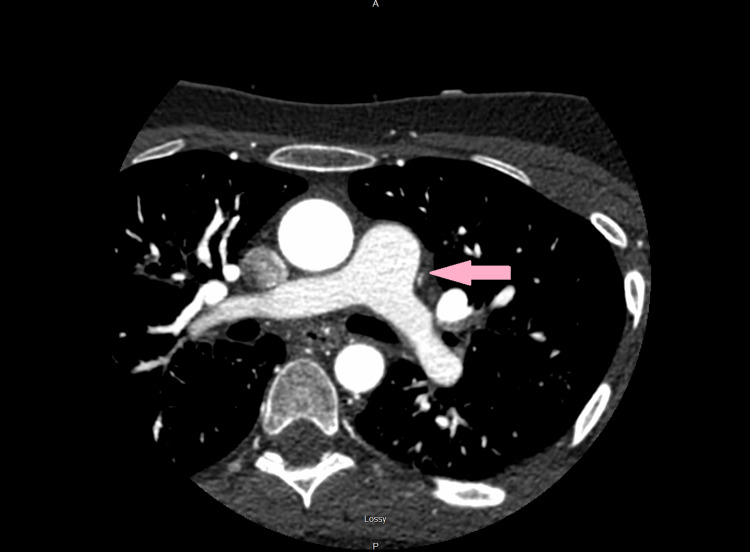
Computed tomography pulmonary angiogram (CTPA) revealing patent pulmonary artery with no signs of pulmonary embolism (pink arrow).
